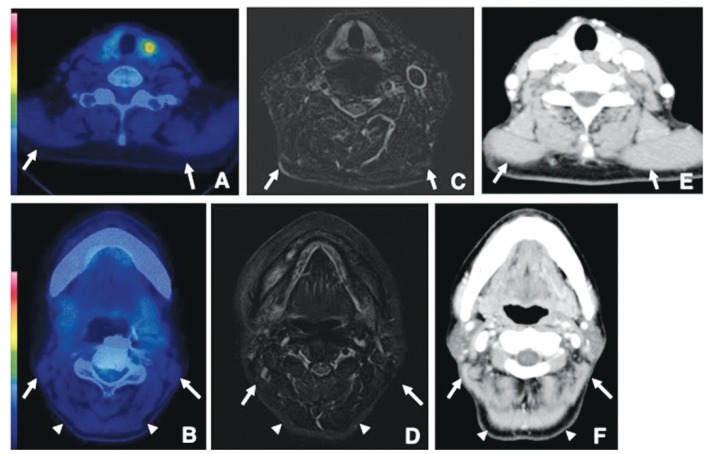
18F-FDG-PET-CT, CT, and MRI examinations of a 61-year-old woman 8 months after surgery without ND for SCC of the right side of the gingiva.
